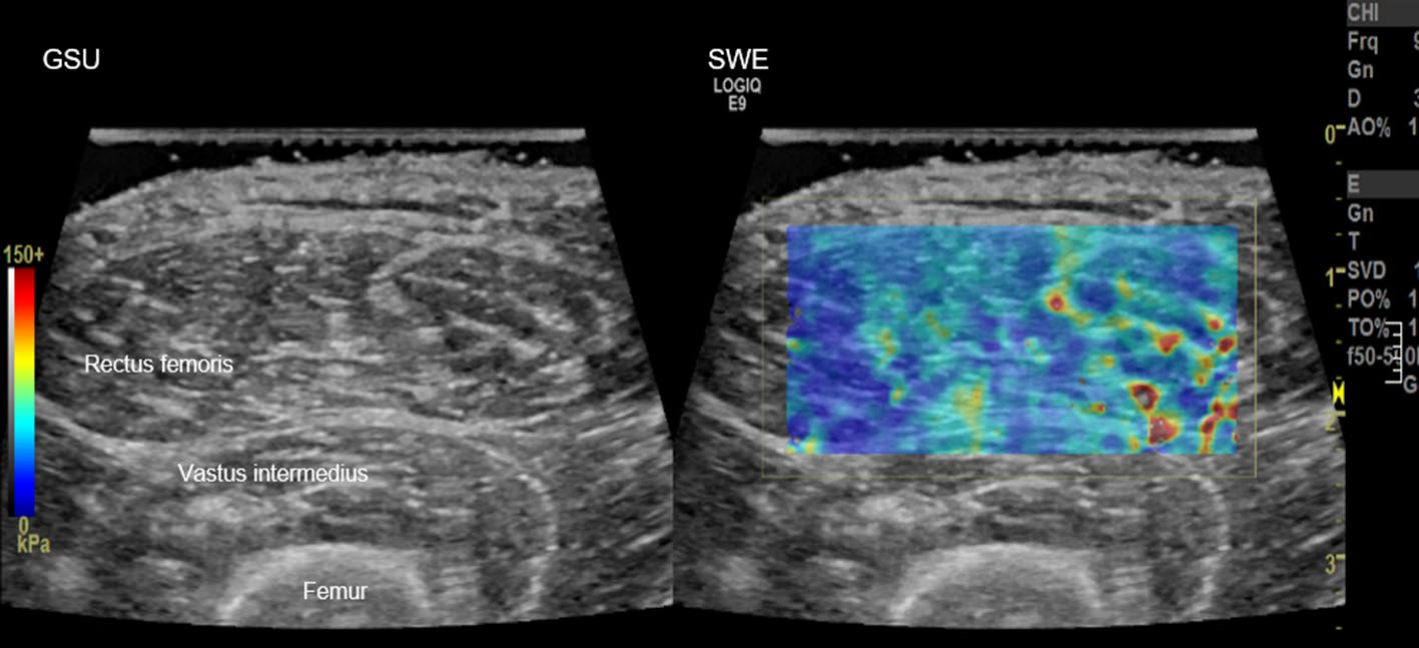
Representative image of ultrasonography.
